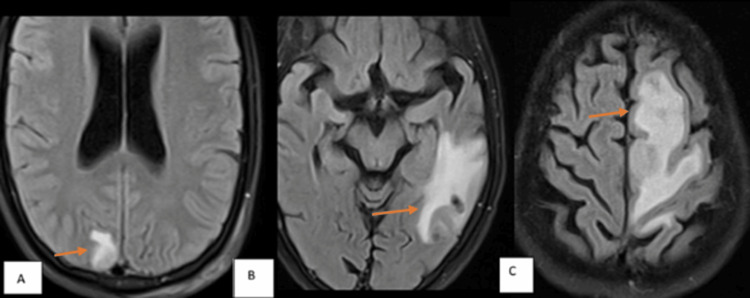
MRI fluid-attenuated inversion recovery (FLAIR) images showing degrees of edema surrounding lesions (A) Case of neurocysticercosis: Lesion in the right occipital lobe (orange arrow) with mild perilesional edema. (B) Case of tuberculoma: Lesion in the left temporal lobe (orange arrow) with moderate perilesional edema. (C) Case of tuberculoma: Lesion in the left high frontal lobe (orange arrow) with severe perilesional edema.
